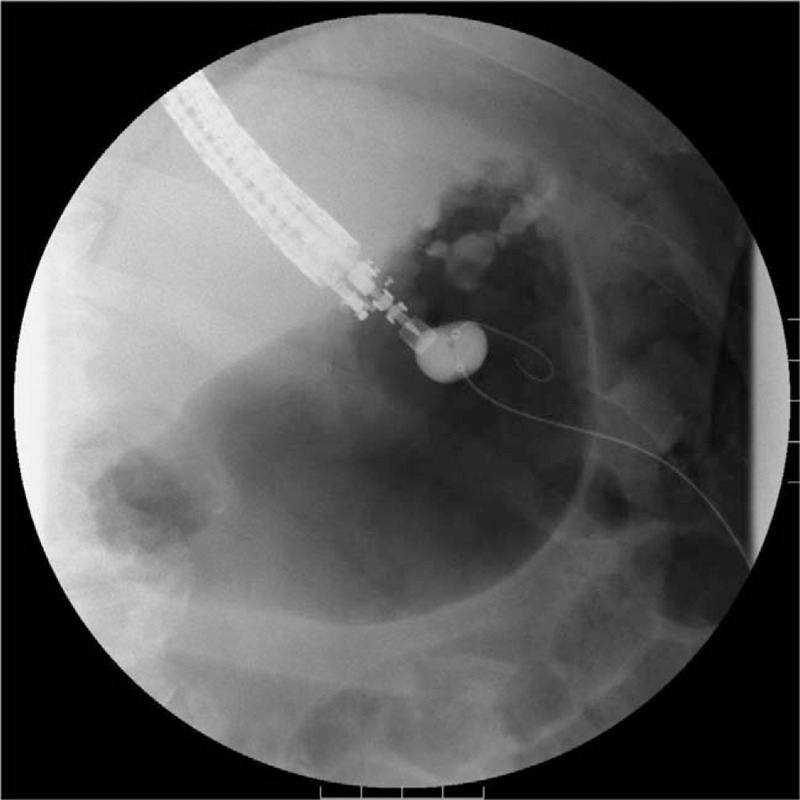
Fluoroscopic image. The balloon-target puncture under endosonographic guidance.
